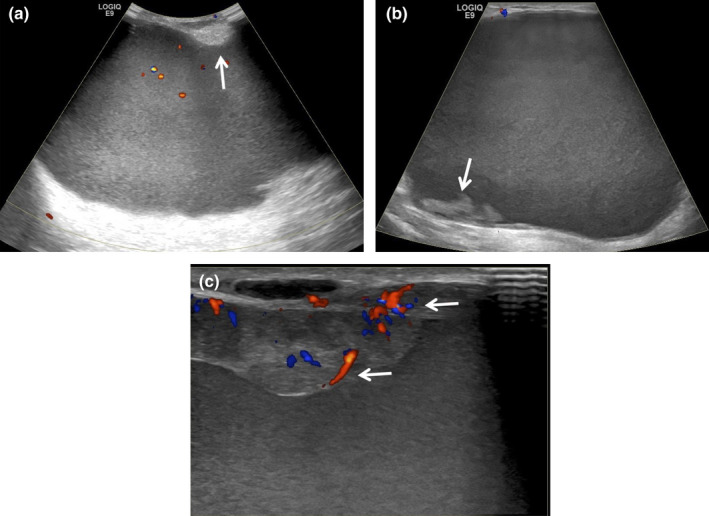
56‐year‐old woman presented to the emergency department due to uncontrolled right breast bleeding. Initial overnight ultrasound images performed with a C1‐6 MHz transducer set to a frequency of 5 MHz by the general sonographer showed a large unilocular cystic lesion with homogeneous internal low‐level echoes and mural nodules (arrow) (a). Repeat imaging 1 day later was done prior to biopsy using a ML6‐15 MHz transducer with the frequency set to 9 MHz in the breast imaging department confirming the large unilocular cystic lesion with irregular margins and homogeneous internal low‐level echoes (b). With reduced manual compression from the transducer and the colour scale set to 5 cm/s, internal vascularity was identified in the mural nodules (arrow) (c). Ultrasound‐guided aspiration of the fluid component and biopsy of the solid component was performed revealing intracystic papillary carcinoma with a low nuclear grade associated with a haematoma.
